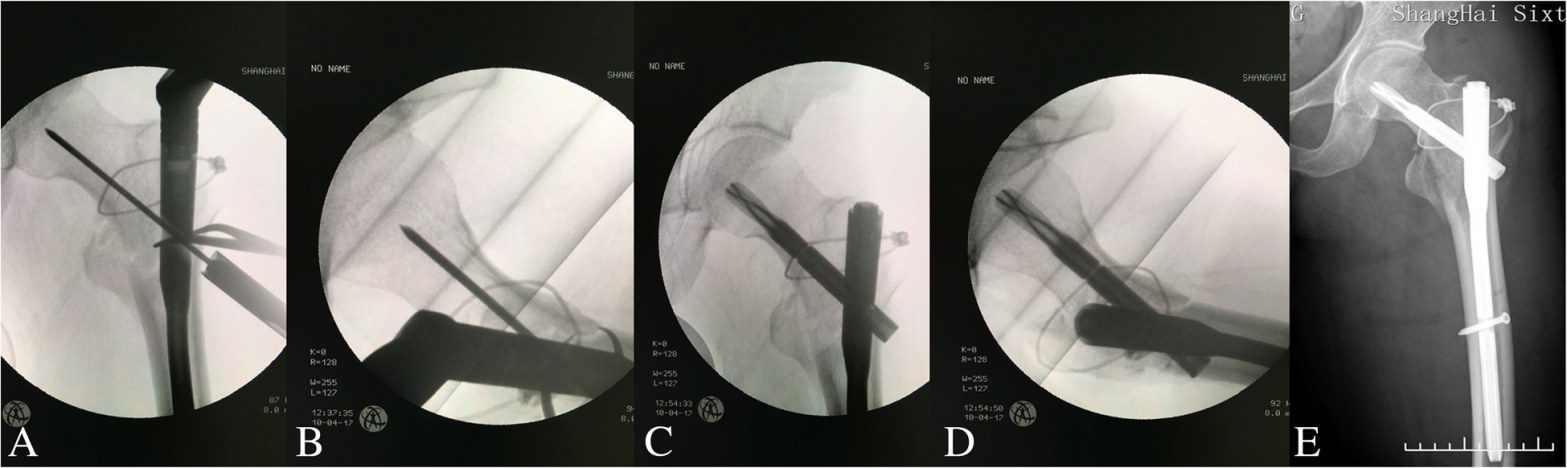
Routine maneuvers of nail fixation. Anteroposterior (a) and lateral (b) imaging show the guide pin for the blade. The pin should be in parallel with the axis of the femoral neck in both planes with correct tip-to-apex distance. Anteroposterior (c) and lateral (d) view shows anatomical reduction and satisfactory fixation by PFN-A. The wire is tightened, and the displaced fragments of the greater trochanter get a better position. e Postoperatively, anteroposterior radiograph shows the perfect combination of the wire and the nail
